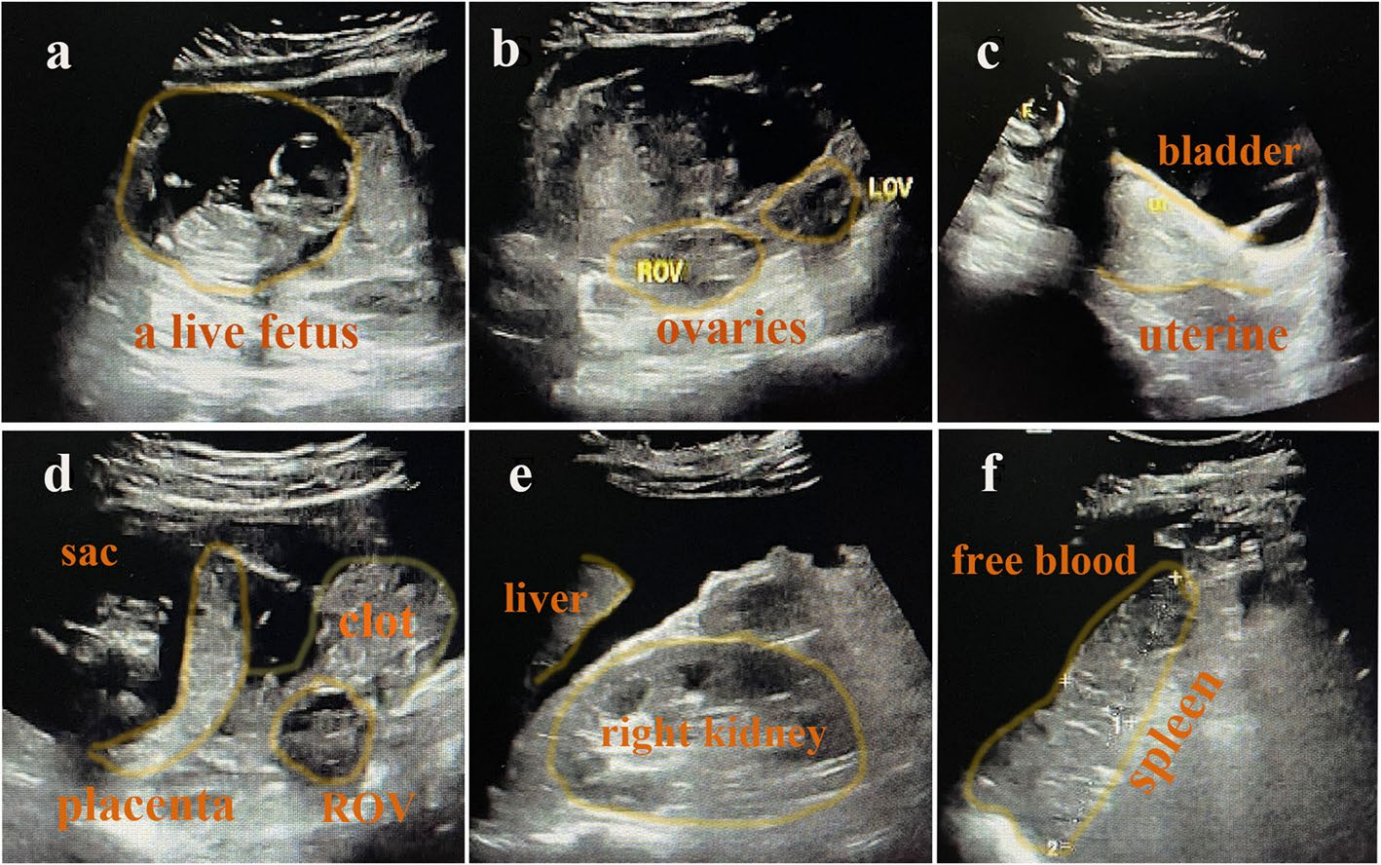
Transabdominal ultrasound images. ROV:right ovary, LOV:left ovary
